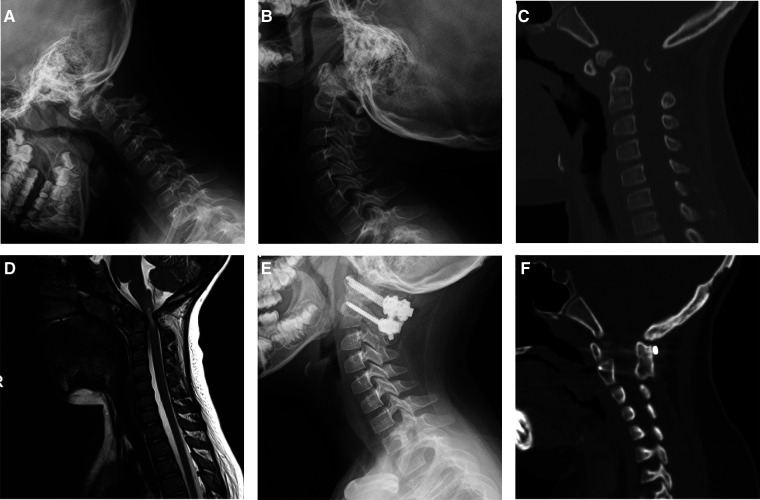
Images of a 8-year-old boy with atlantoaxial dislocation due to os odontoideum. This patient underwent atlantoaxial fusion with the use of OBG. Preoperative plain radiograph in flexion (A), extension (B) showed atlantoaxial dislocation. CT showed os odontoideum (C). MRI showed neural element compression (D). Postoperative plain radiograph in neutral (E) showed rigid fixation. Computed tomography sagittal reconstruction showed solid bony fusion 12 months after surgery, white arrow showed occipital bone donor-site (F).
